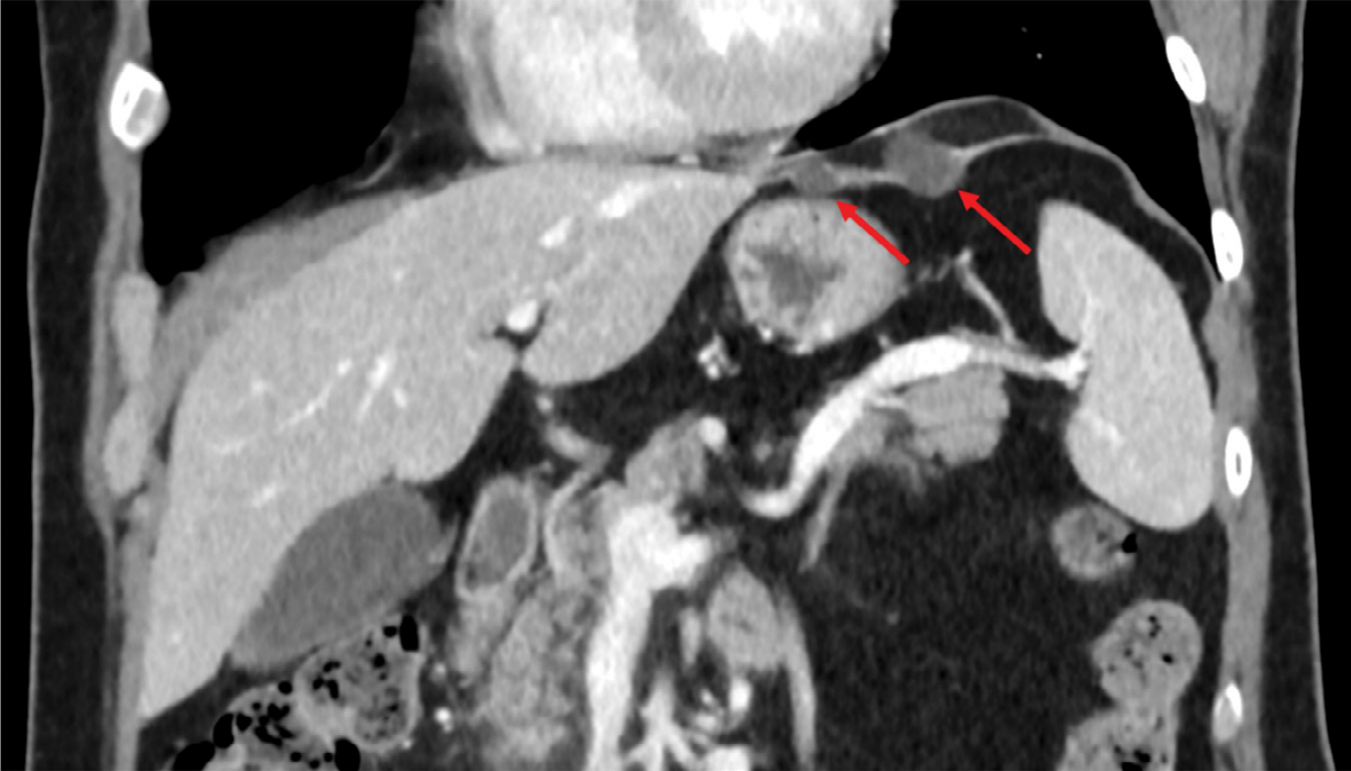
Corional view of the contrast enhanced abdominal CT‑scan showing the cystic lesions (arrows).
